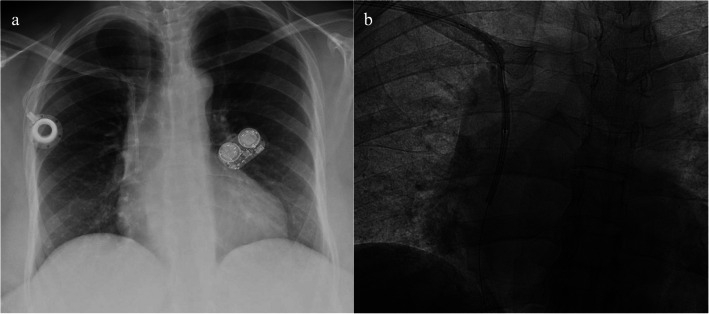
a: preoperative PA chest x-ray demonstrating right-sided chest port with a subclavian tunneled catheter and pericatheter calcifications along its course through the superior vena cava; b: intraoperative fluoroscopy demonstrating angioplasty with a 3 mm balloon as it disrupts the calcified adhesions to allow for removal of the catheter in its entirety without fragmentation
